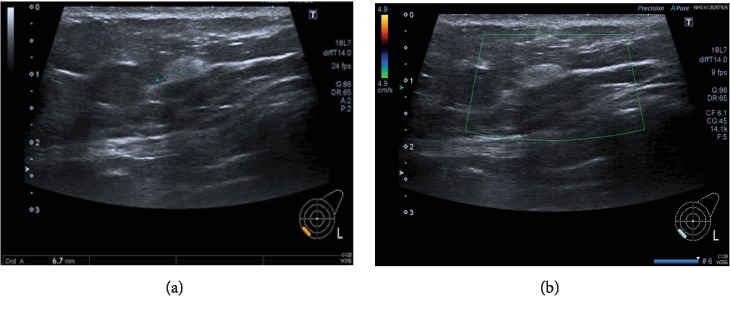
These ultrasound images show a 6.7 mm, well-defined ovoid echogenic lesion, in the lower inner quadrant (U2/3). Three 14-gauge needle core samples were taken.
